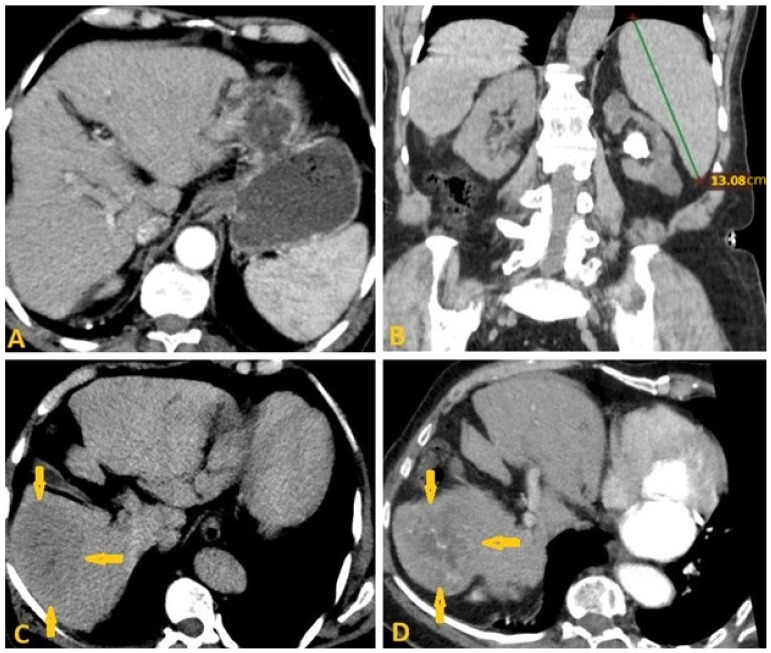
Selected computed tomography images (same patient as in Figure 6: axial (A,C,D) and coronal reconstruction (B) of a 79-year-old man show irregular borders of the liver with widening interlobar fissure in keeping with chronic liver disease (A,B). In addition, there is a 75 × 57 mm ill-defined hypodense mass in segment-6 (arrows on (C)), revealing early heterogeneous enhancement after contrast administration (arrows on (D)) that rapidly washed out in the delayed phase, which is consistent with hepatocellular carcinoma complicated by liver cirrhosis as a result of chronic Schistosomiasis.
